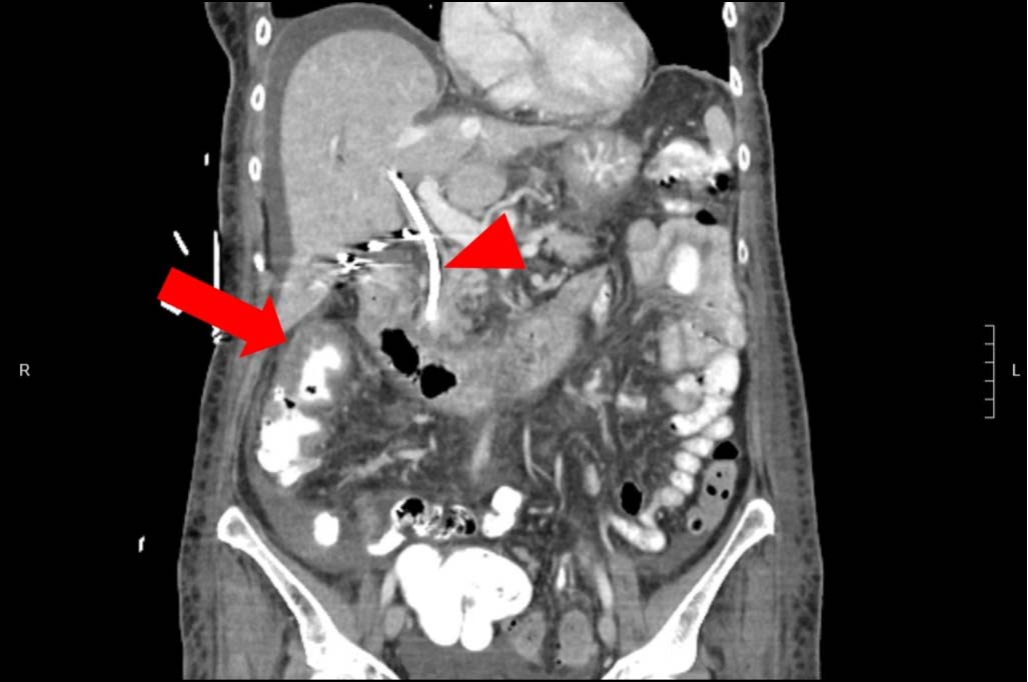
Abdominal and pelvic computed tomography with intravenous and oral contrast showing segmental wall thickening of the ascending colon (arrow) consistent with colitis. The biliary stent is also visualized (arrowhead).
